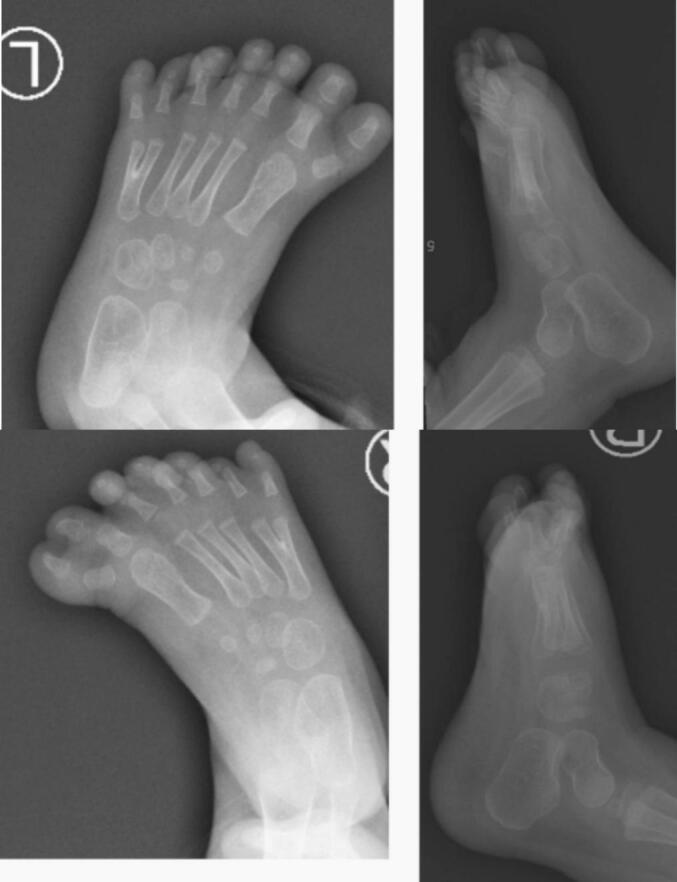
Anteroposterior and lateral radiographic examination of the left and right foot revealed a bifid fifth metatarsal.
